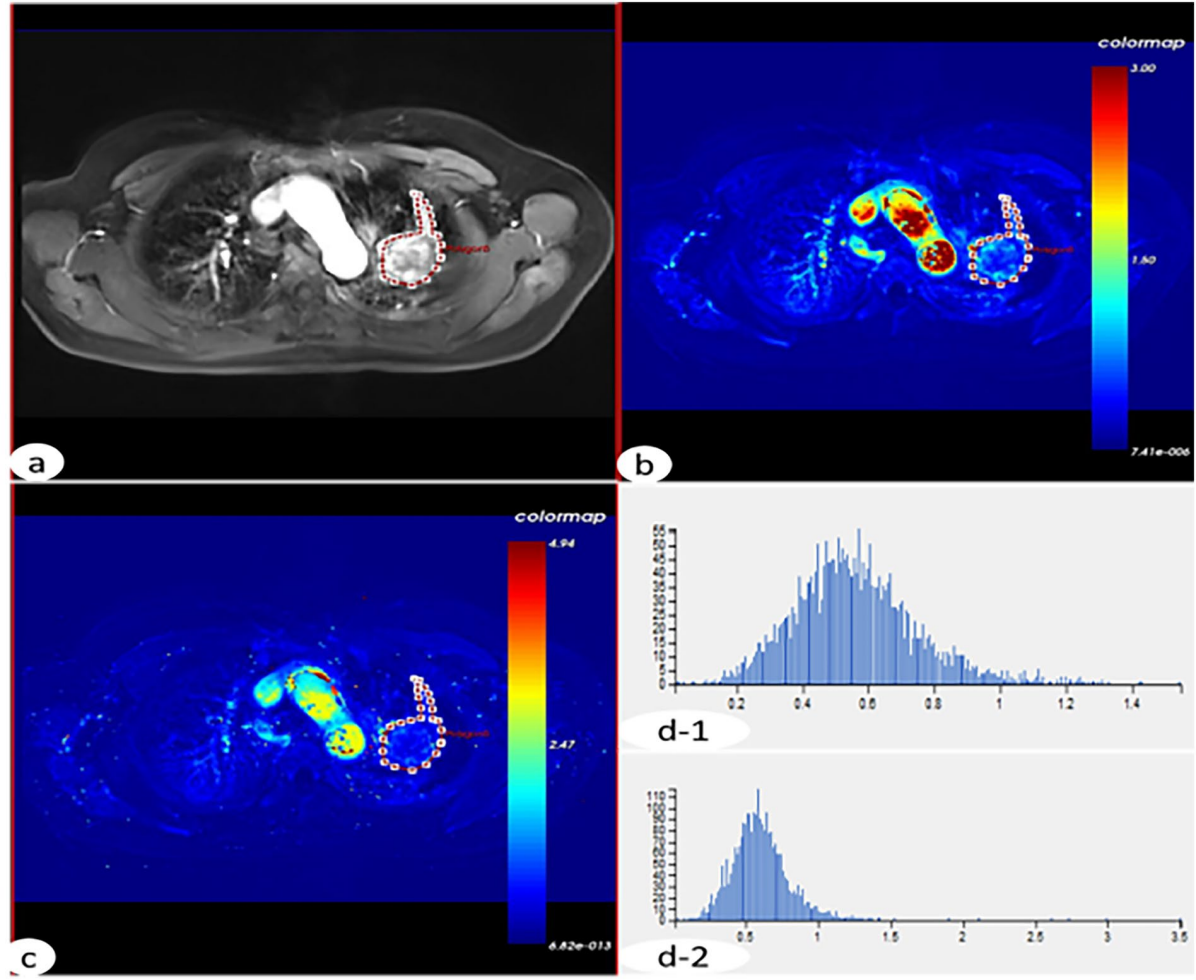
A 52-year-old female, was pathologically diagnosed as adenocarcinoma of the left upper lung. (a) The original DCE-MR image of the patient’s chest, and the red circle area is the focus; (b) the F_p_ image of the corresponding EC model, and the red circle area is the focus; (c) the corresponding EC model KTrans image, and the red circle area is the focus. (d-1) The histogram of the Fp perfusion parameters of the EC model (mean = 0.5639; homogeneity = 0.6543; skewness = 0.6992; kurtosis = 1.2016; energy = 0.0111; entropy = 6.7617). (d-2) The histogram of the KTrans perfusion parameters of the EC model of the lesion (mean = 0.6077; uniformity = 0.6358; skewness = 2.5321; kurtosis = 22.0993; entropy = 5. 7501; energy = 0.0229).
